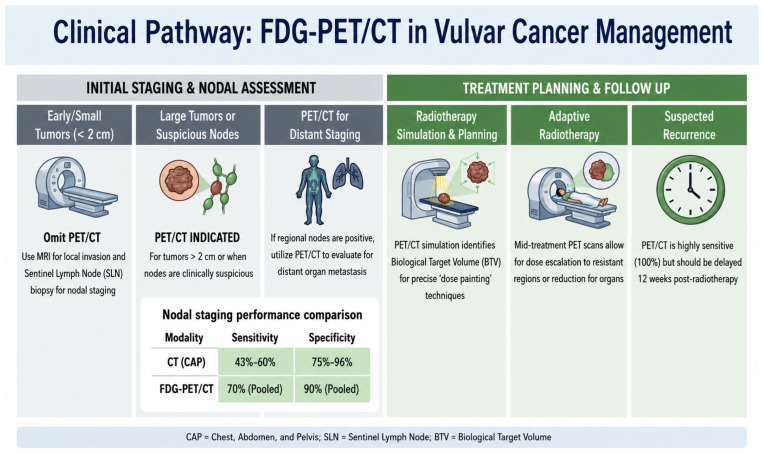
Clinical algorithm for the use of FDG PET/CT in vulvar cancer.
